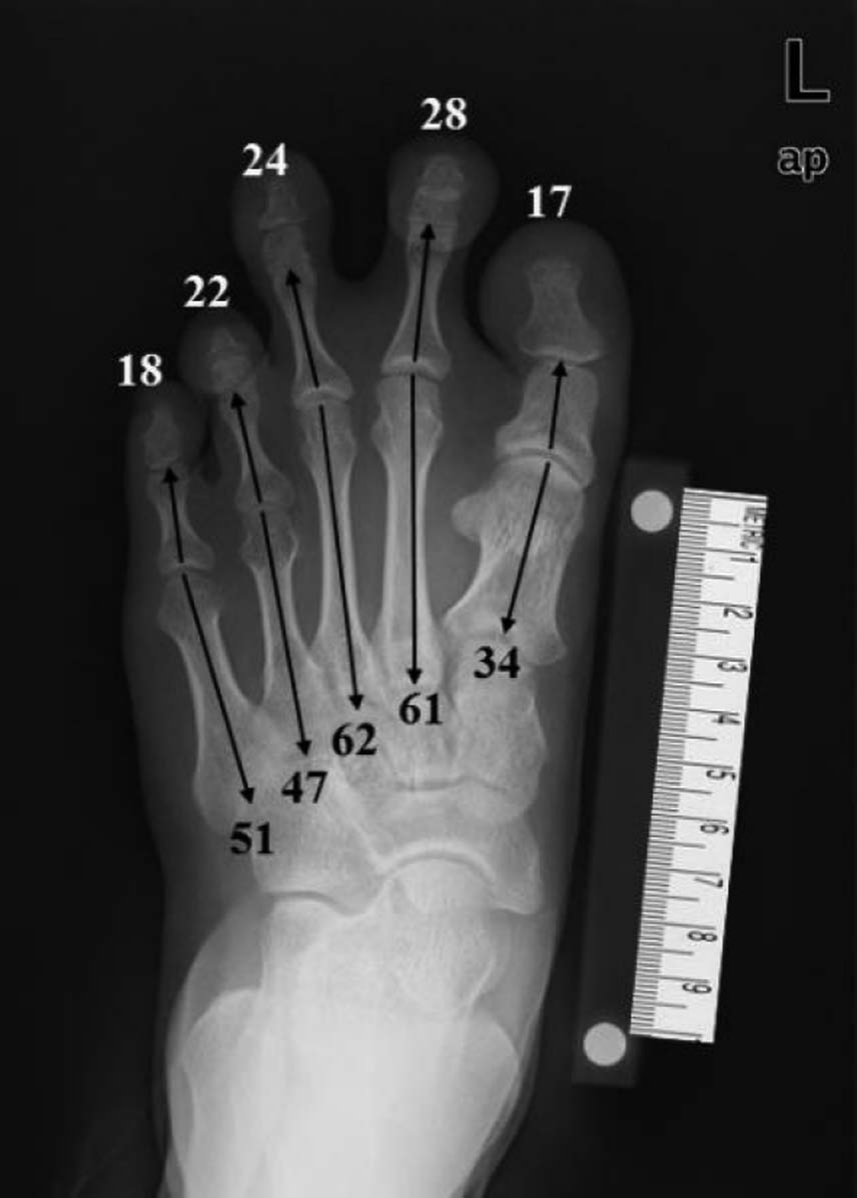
Anteroposterior radiograph of the foot, showing brachymetatarsia in the first, fourth, and fifth rays. The numerical values represent the lengths (in millimeters) of the metatarsals (black numbers) and the phalanges (white numbers).
